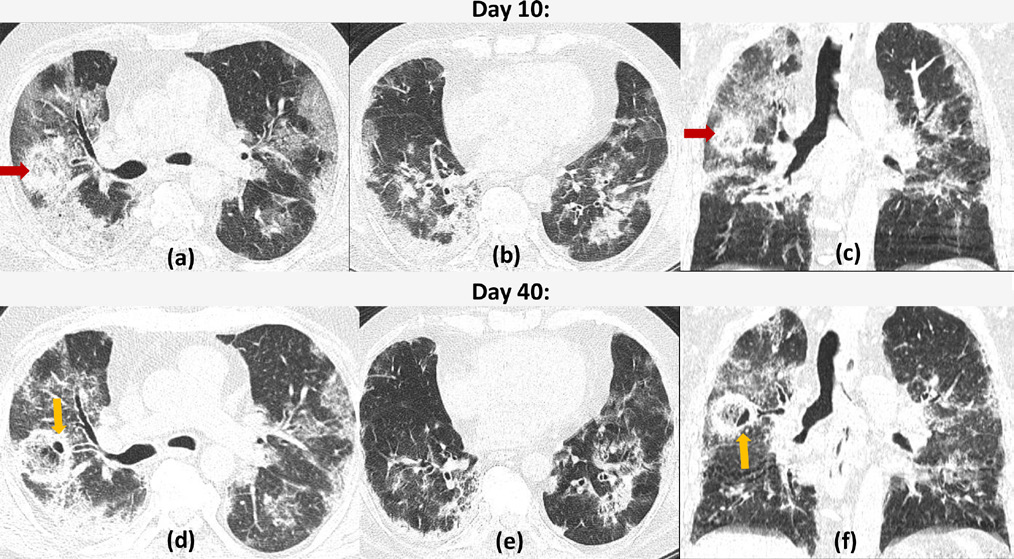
COVID19 with cavitation and *Atoll sign:* A 45-years-old COVID19 positive patient on 10th day of illness. Axial and coronal reformatted CT sections (a–c) show multifocal ground glass opacities and consolidations with a basal and peripheral predominance. A spherical area of central ground glass opacity and peripheral consolidation s/o *Atoll sign* can be seen in the right upper lobe (red arrow). Serial CT (d–f) done 1 month later revealed development of a few small cavitatory lesions (yellow arrows) both in the peripheral rim of consolidation as well as in the central area of ground glass opacities of the right upper lobe lesion. The other peripheral ground glass opacities had slightly reduced and had become more organized.
